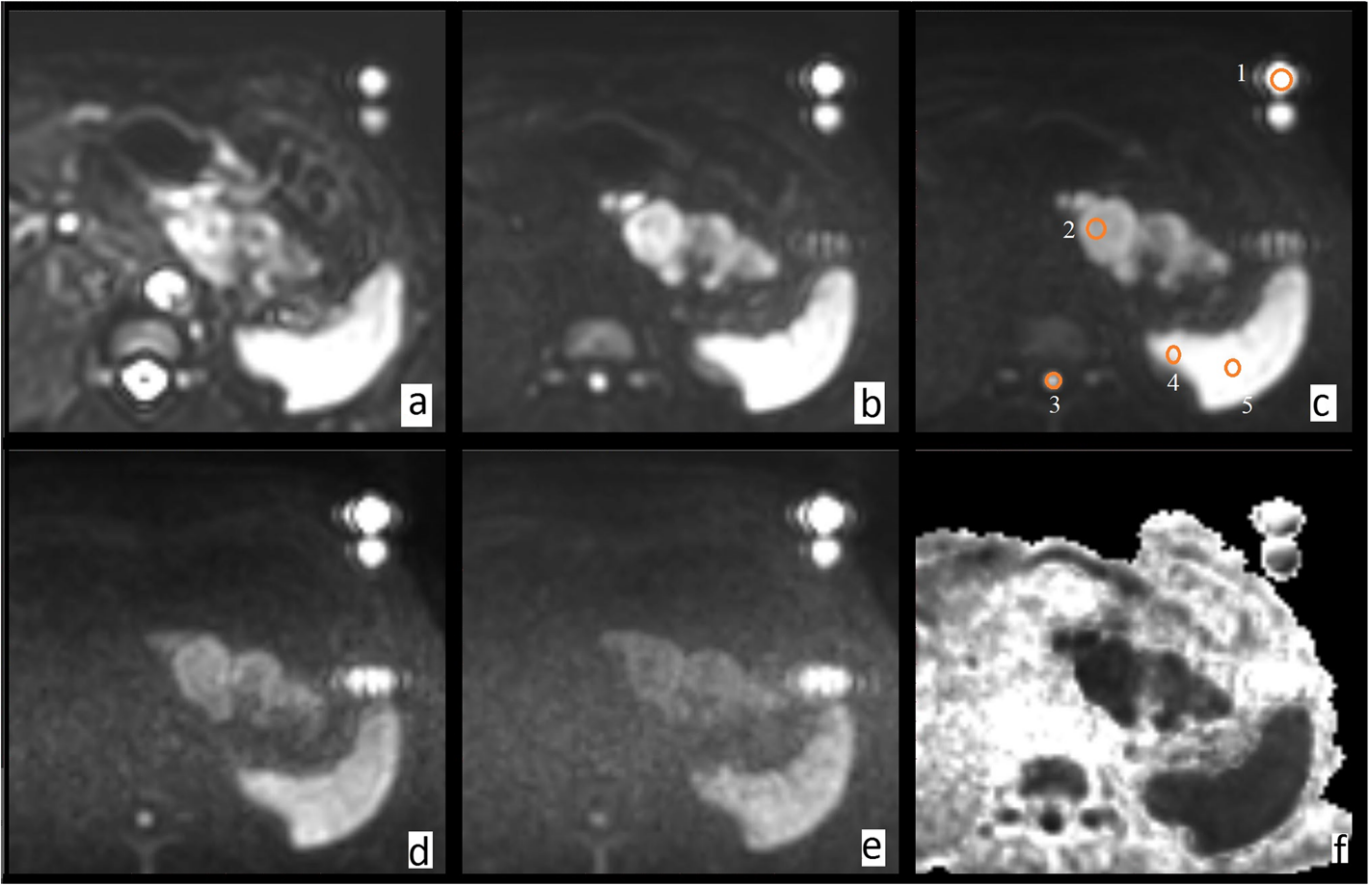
The in vivo DWI with *b*-factor of (a) 0 s/mm^2^, (b) 500 s/mm^2^, (c) 1000 s/mm^2^, (d) 2000 s/mm^2^, (e) 3000 s/mm^2^, and (f) exponential image
